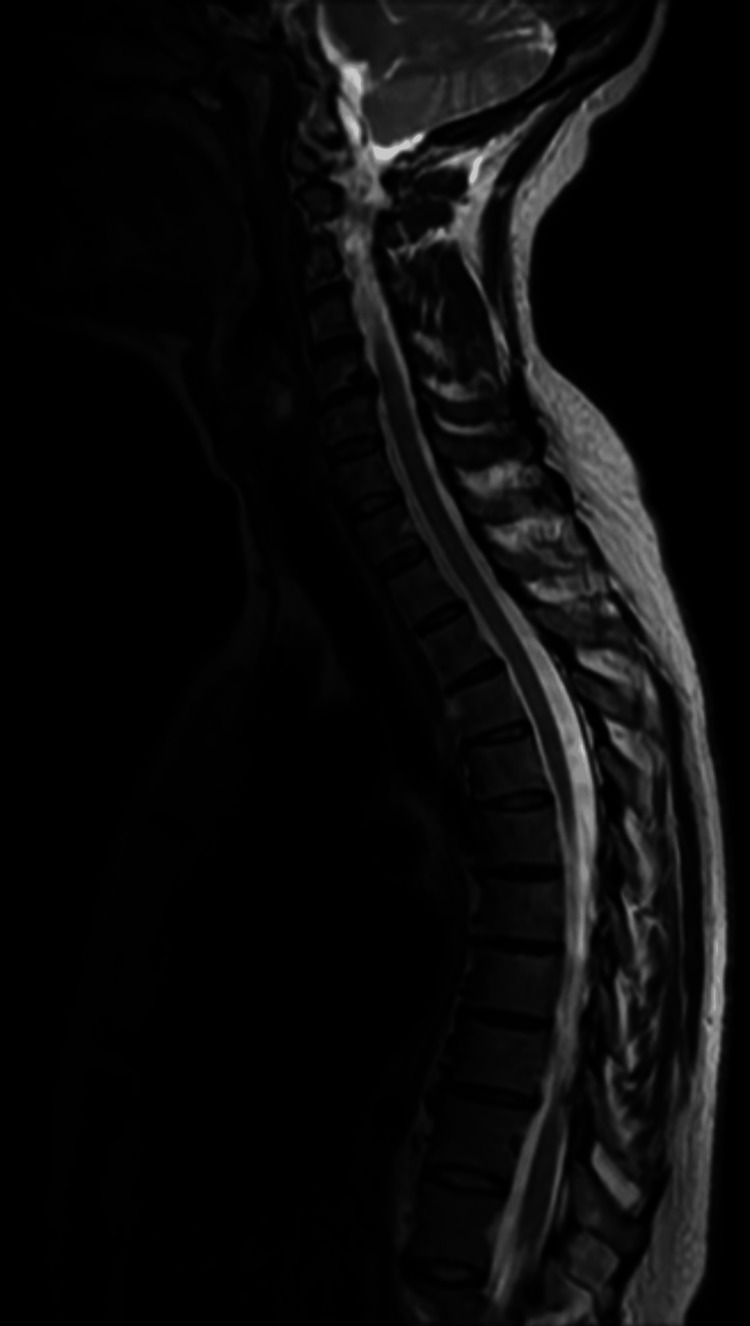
Preoperative sagittal T2-weighted MRI.
